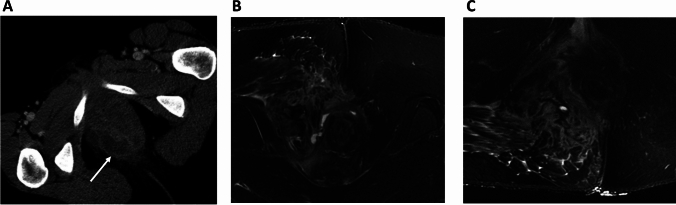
A computed tomography (CT) scan can be used in emergency setting to diagnose pelvic abscesses and to determine the presence and extent of pelvic sepsis. CT-scan of a 37 year old female with Crohn’s disease with clinical complaint of a perianal abscess. A CT-scan in emergency setting confirmed perianal abscess (arrow). B, C Follow-up postoperative MRI one day after surgical treatment. Resolution of the abscess after incision and drainage and small perianal fistula tracts remain visible. Furthermore, MR-images depict tissue inflammation surrounding the fistula tract
